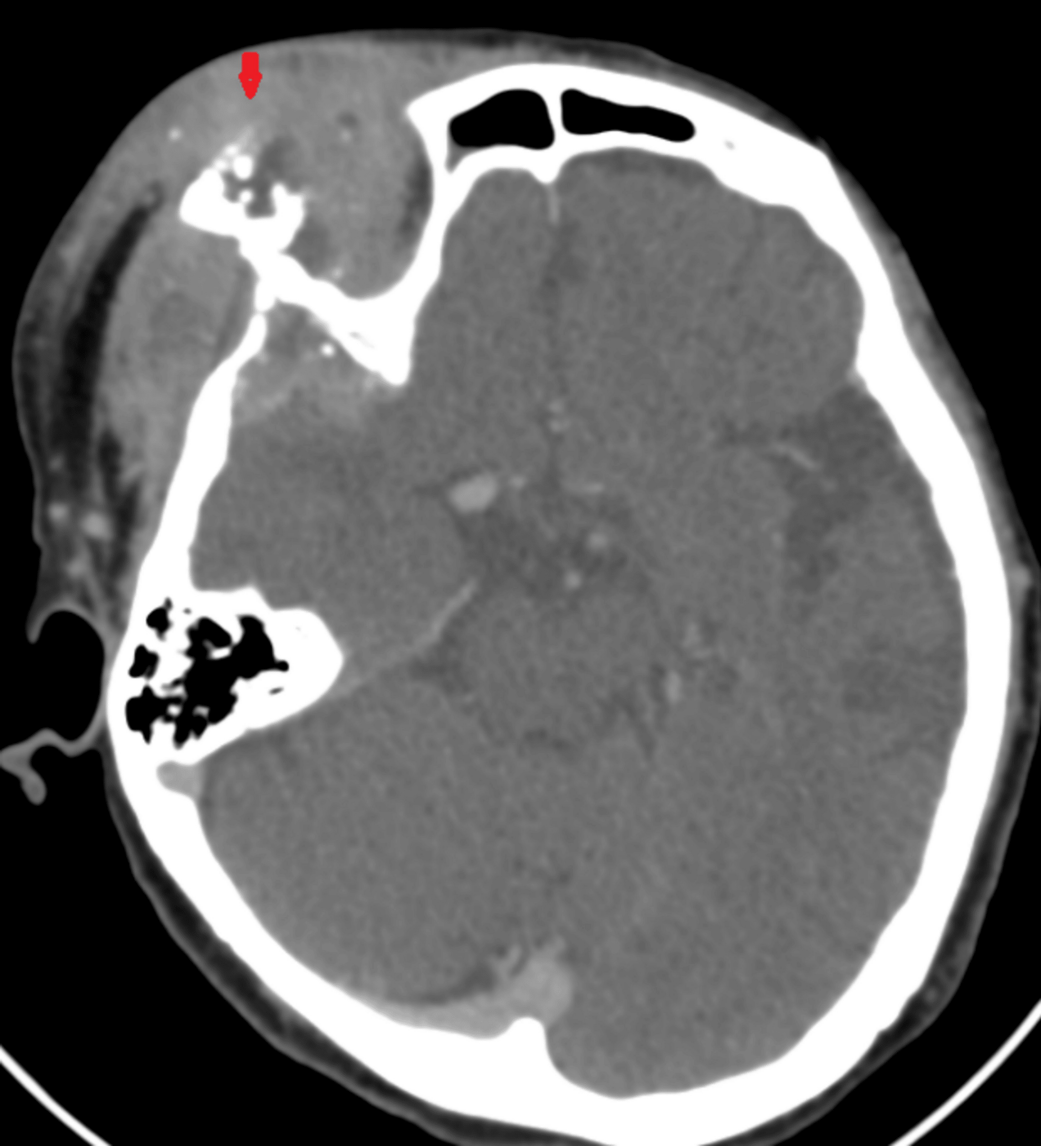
CT orbit and brain (axial view) on the third presentation CT: computed tomography
